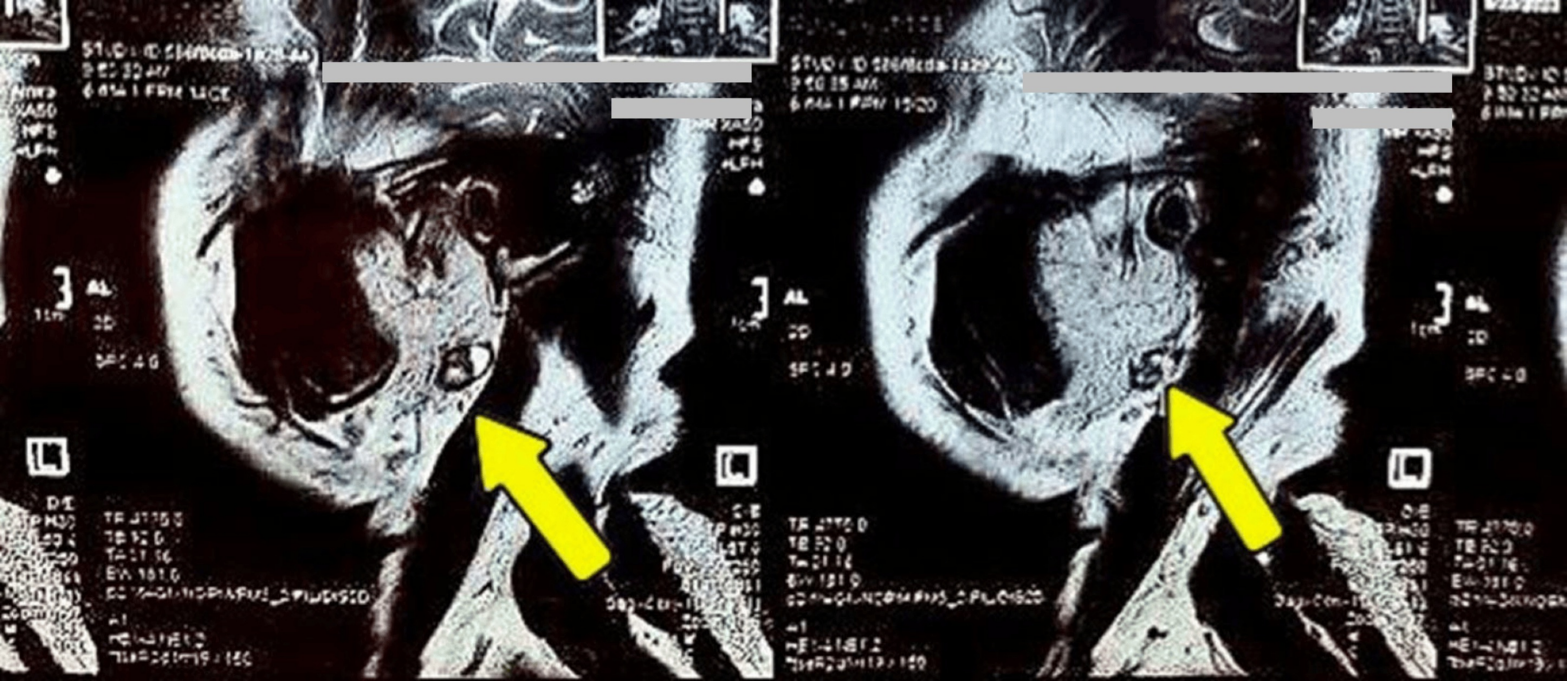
A preoperative MRI scan of the patient The figure shows two different sections of the sagittal view of the patient. The arrow shows the presence of a well-evident single ovoid mixed hyper-intense and hypo-intense area with well-defined margins in the superficial lobe of the left parotid gland.
